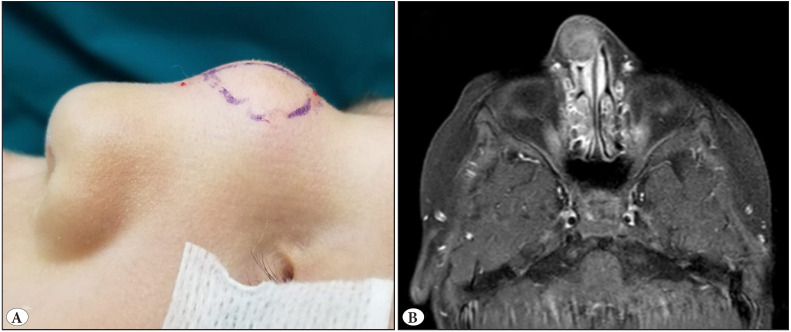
A) The external physical examination revealed a 2 cm-sized mass on the nasal dorsum. B) T1-weighted MRI shows a 2.2x1.9x1.6 cm-sized, hypointense, well-circumscribed, nodular solid mass on the tip of the nose.
